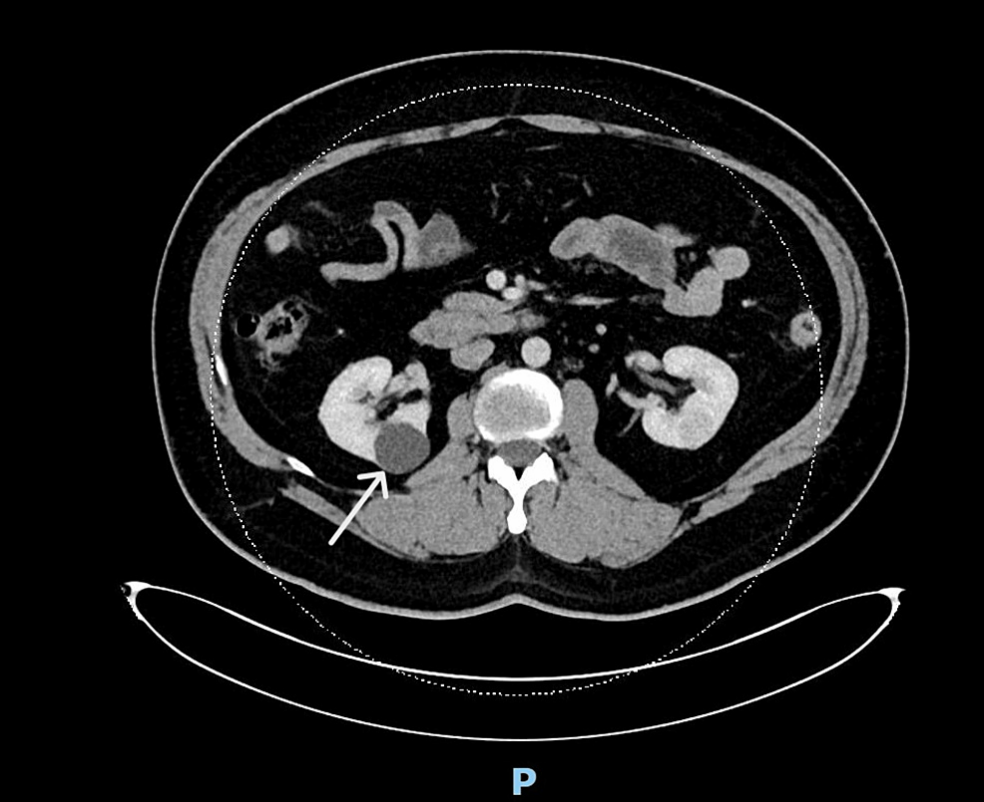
CT scan of the abdomen showing homogeneously enhancing, well-circumscribed, round lesion (arrow) in the interpolar region of the left kidney. CT, computed tomography
